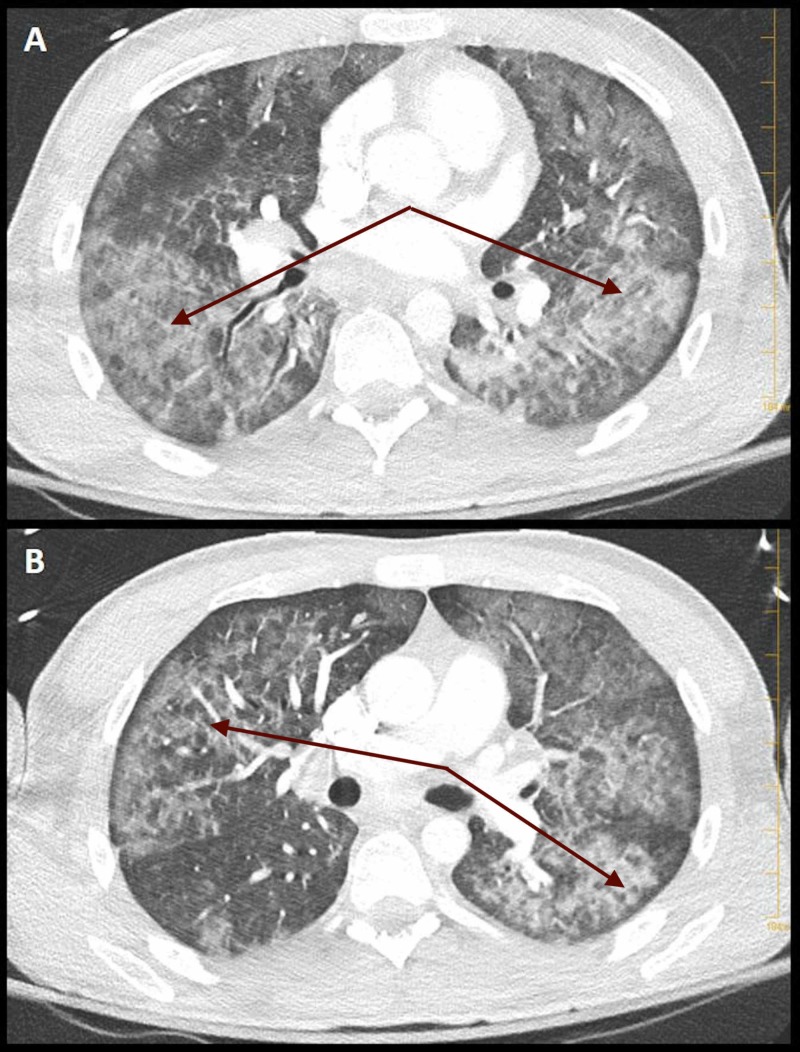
Computed tomography of the chest. (A, B) Axial section of computed tomography of the chest (arrows) demonstrating bilateral ground-glass opacities.
